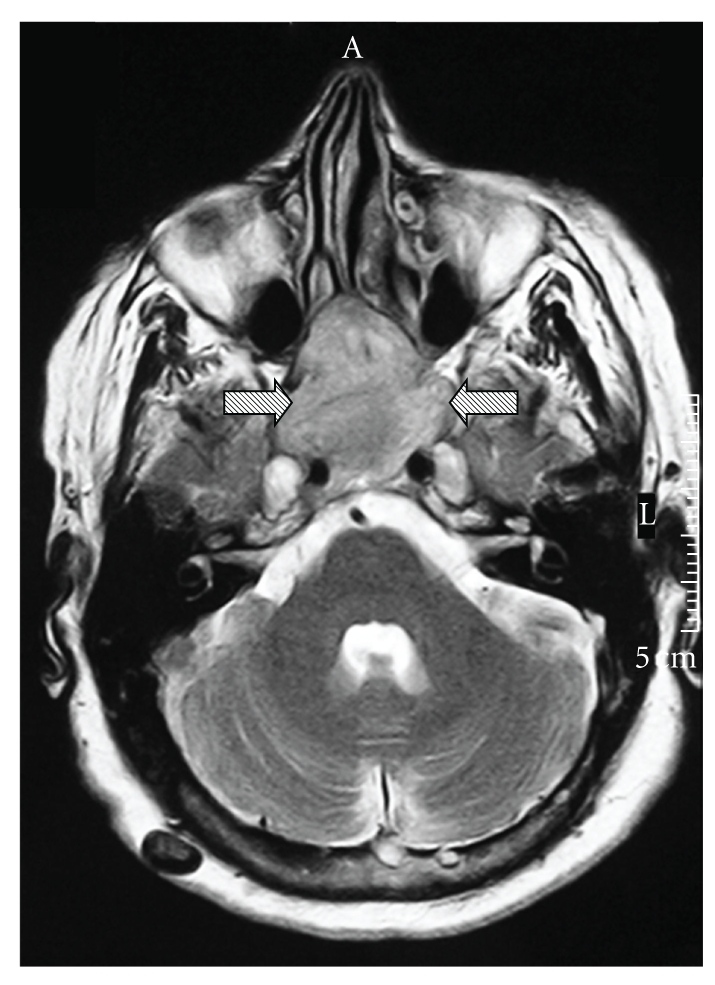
MRI image: a large soft tissue mass (arrows) invades the skull base.
